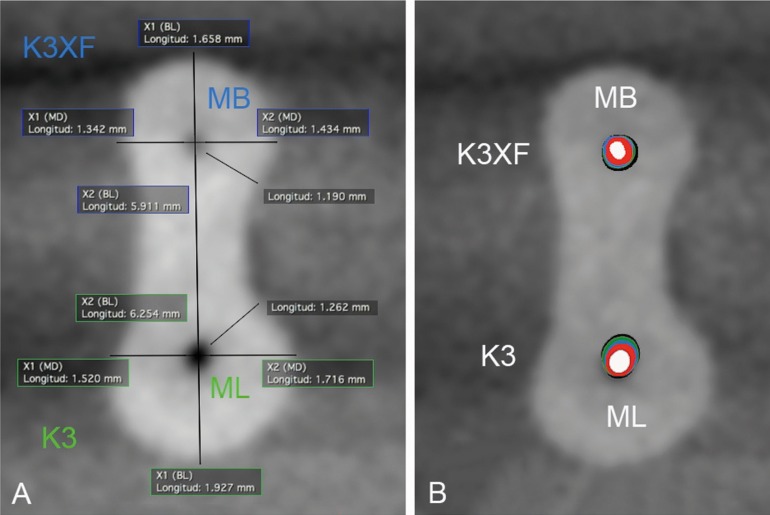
A) An example of measurements obtained from the CBCT images for measurement of canal transportation, centering ability and dentin removal toward the danger zone. B) An example of a CBCT section showing the original canal in white and the prepared outline in red (25.04), blue (30.04), green (35.04), black (40.04). MB canal prepared with K3XF instruments and ML canal with K3 instruments.
